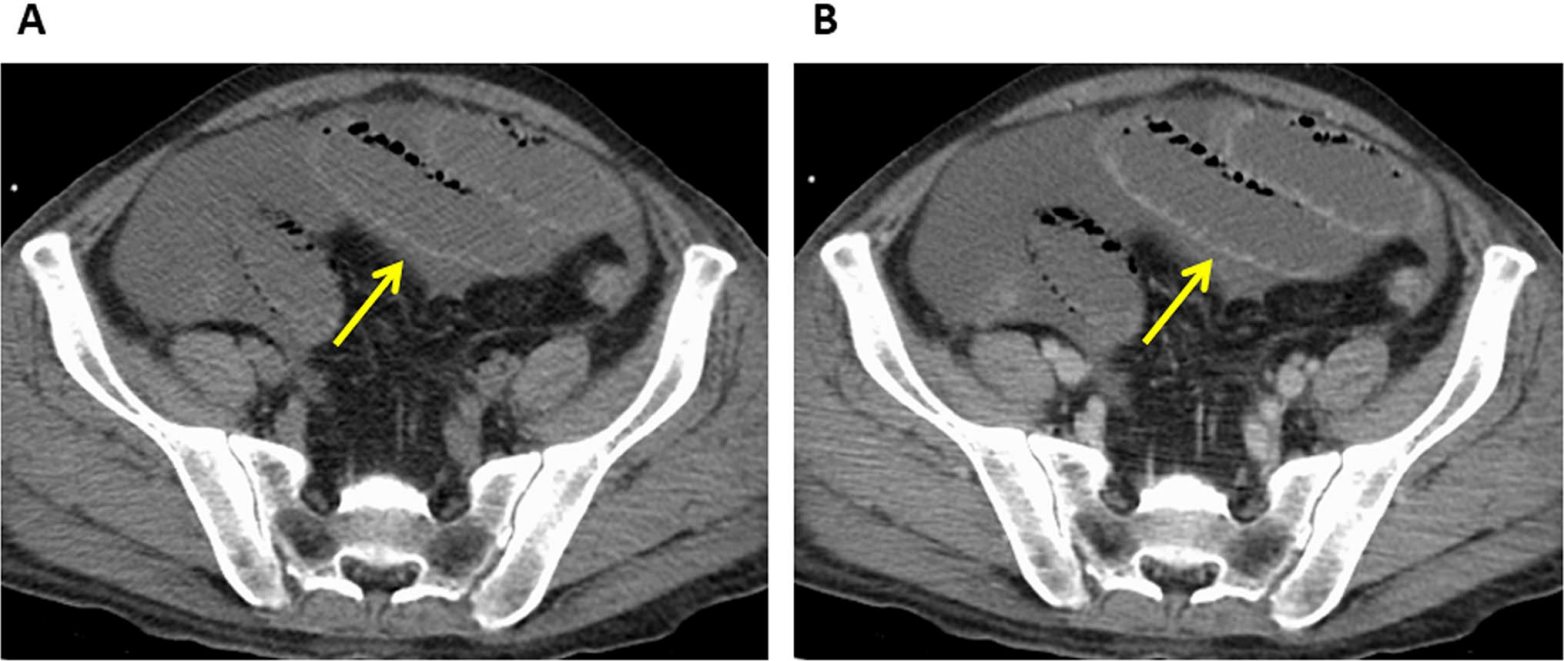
Non-occlusive mesenteric ischemia in a 50-year-old man with a history of alcoholic liver failure. A. Unenhanced CT image. The bowel wall is highly attenuated (arrow). B. Contrast-enhanced CT image. The wall seems to be well enhanced due to high attenuation (arrow). The unenhanced and enhanced images must be compared for an accurate assessment of bowel-wall contrast enhancement
